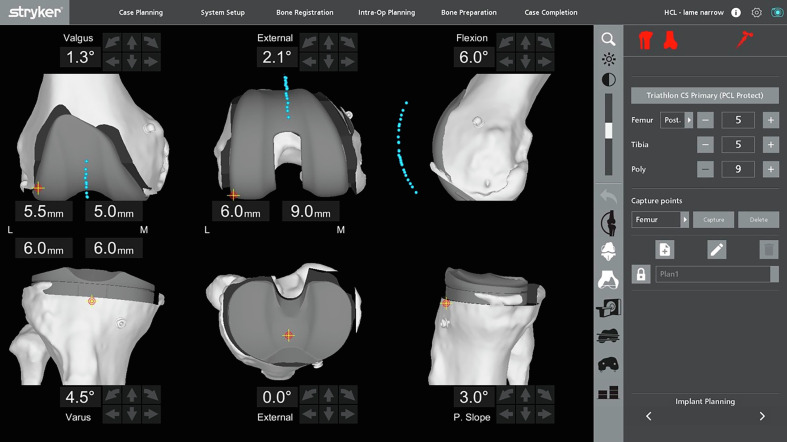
Patellar tracking before the bone resection on the CT scan of the knee planning.
